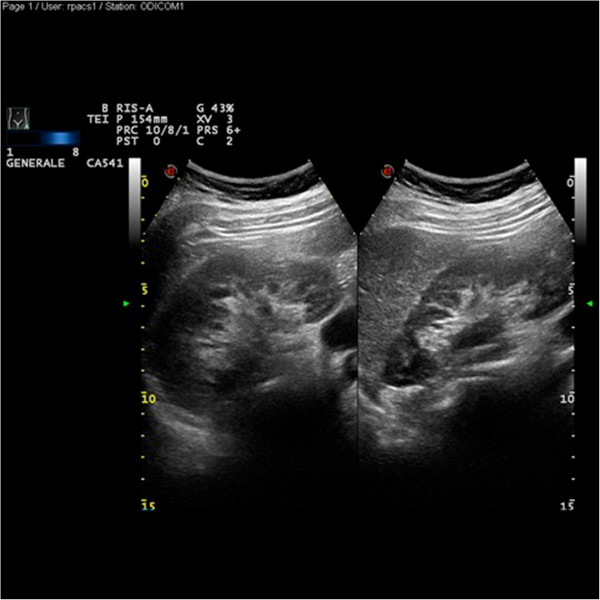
Kidney ultrasounds in case 5, after the positioning of JJ stent. Only minimal dilatation is present; however, proteinuria persisted after “JJ stenting”, reducing the dilatation, and in the absence of infection. “JJ” was removed one week after delivery (proteinuria 0.5 g/day); proteinuria was absent at the control one month after delivery.
